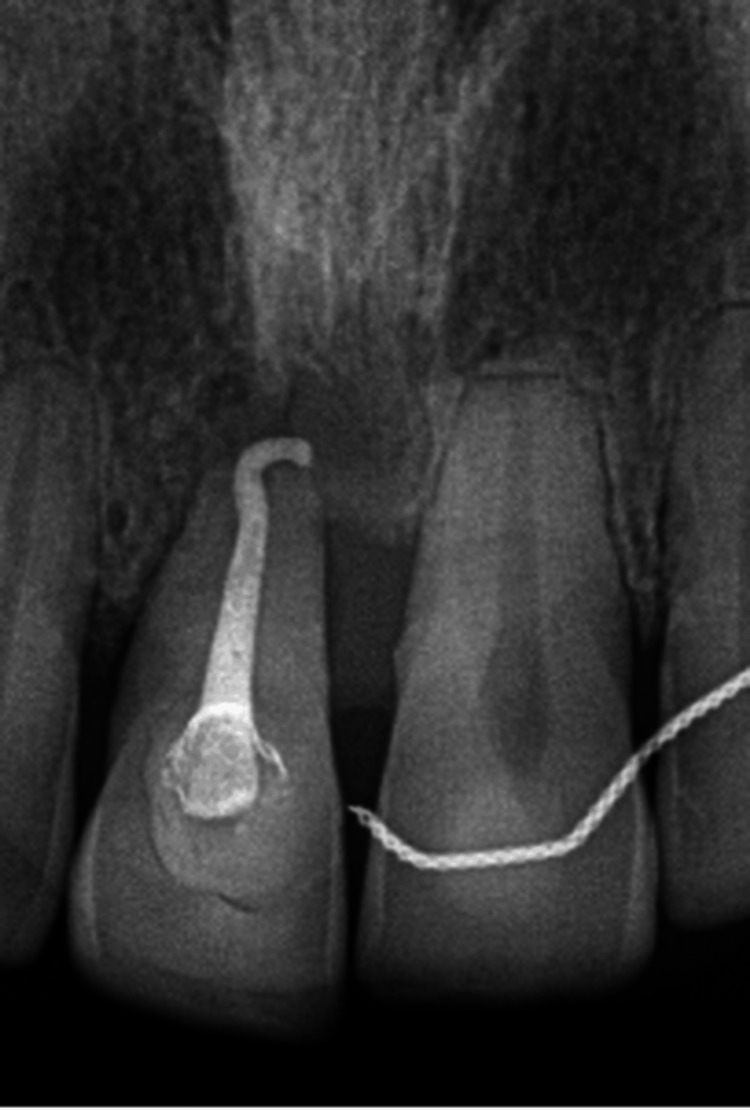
Intraoral periapical radiograph showing root canal treatment of tooth 11 with apical external root resorption
